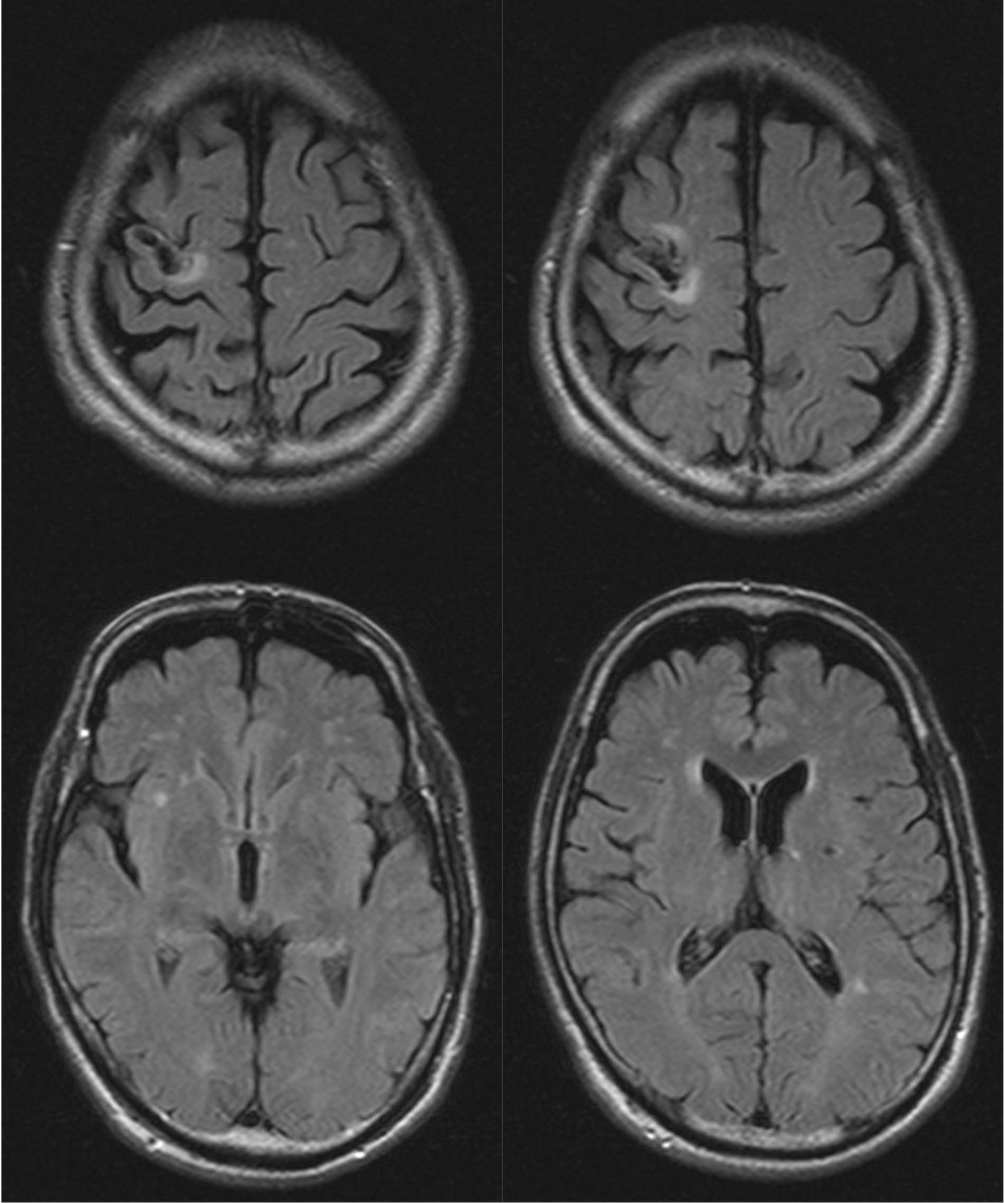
Fluid-attenuated inversion recovery (FLAIR) images of the patient’s brain.
